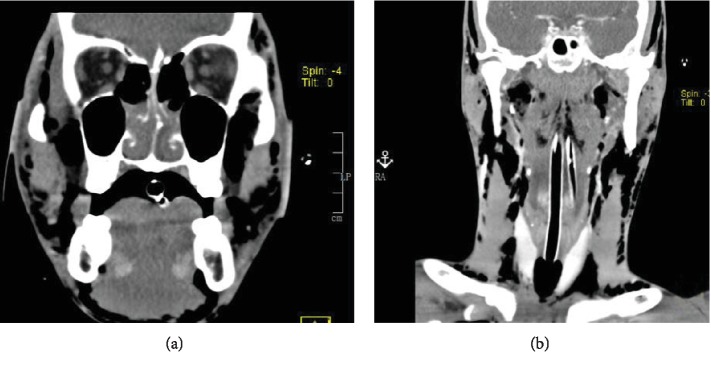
(a) Head computed tomography (CT) coronal view showing extension of subcutaneous emphysema into the orbits and superficial bitemporal soft tissues. (b) Neck computed tomography (CT) coronal view showing massive soft tissue emphysema enveloping the strap muscles of the upper chest, neck, and deep facial spaces of the neck.
